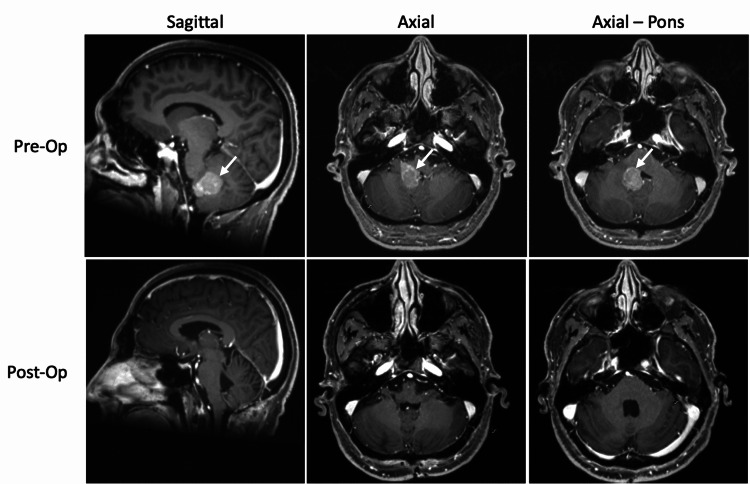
Pre- and postoperative T1-weighted gadolinium-enhanced MRI images in sagittal and axial planes. Preoperative T1-weighted MRI reveals a 2.6 cm enhancing fourth ventricular mass (white arrows), with extension to the right foramen of Luschka. There is a mass effect on the ipsilateral medulla without evidence of obstructive hydrocephalus. Postoperative MRI demonstrates image complete, gross-total resection of the tumor.
